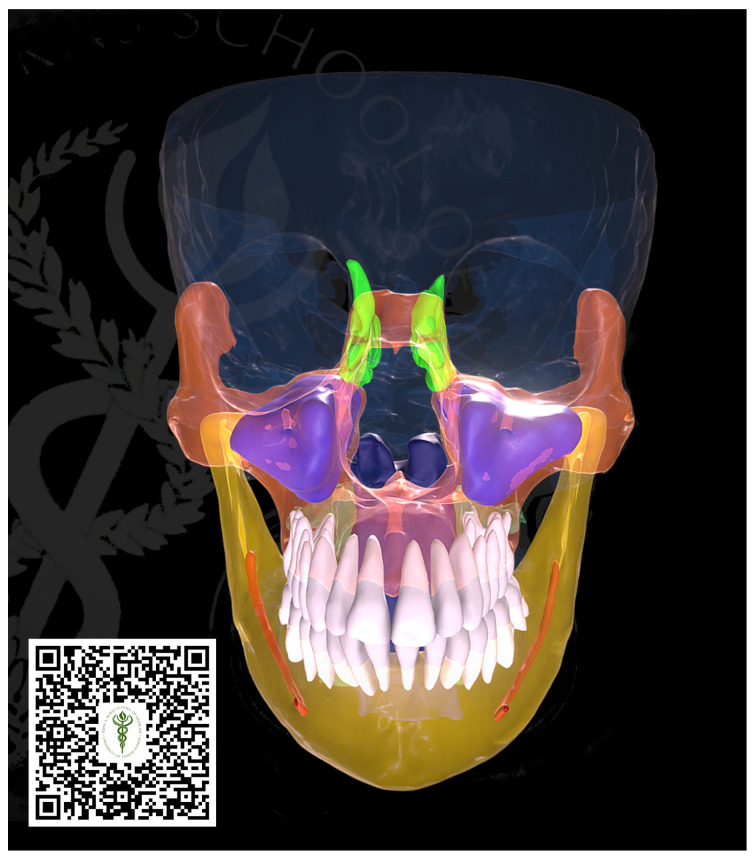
Three-dimensional surface model for visualization. The model presents some radiographic findings such as aplastic frontal and sphenoid sinuses and hypoplastic ethmoid sinuses. By scanning the barcode below using a phone camera, the 3D surface model can be manipulated on the phone screen.
